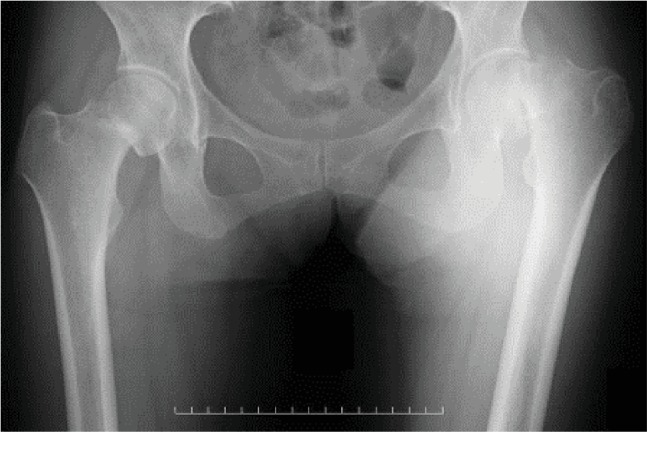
Radiograph of the hip 12 months after TKA.
